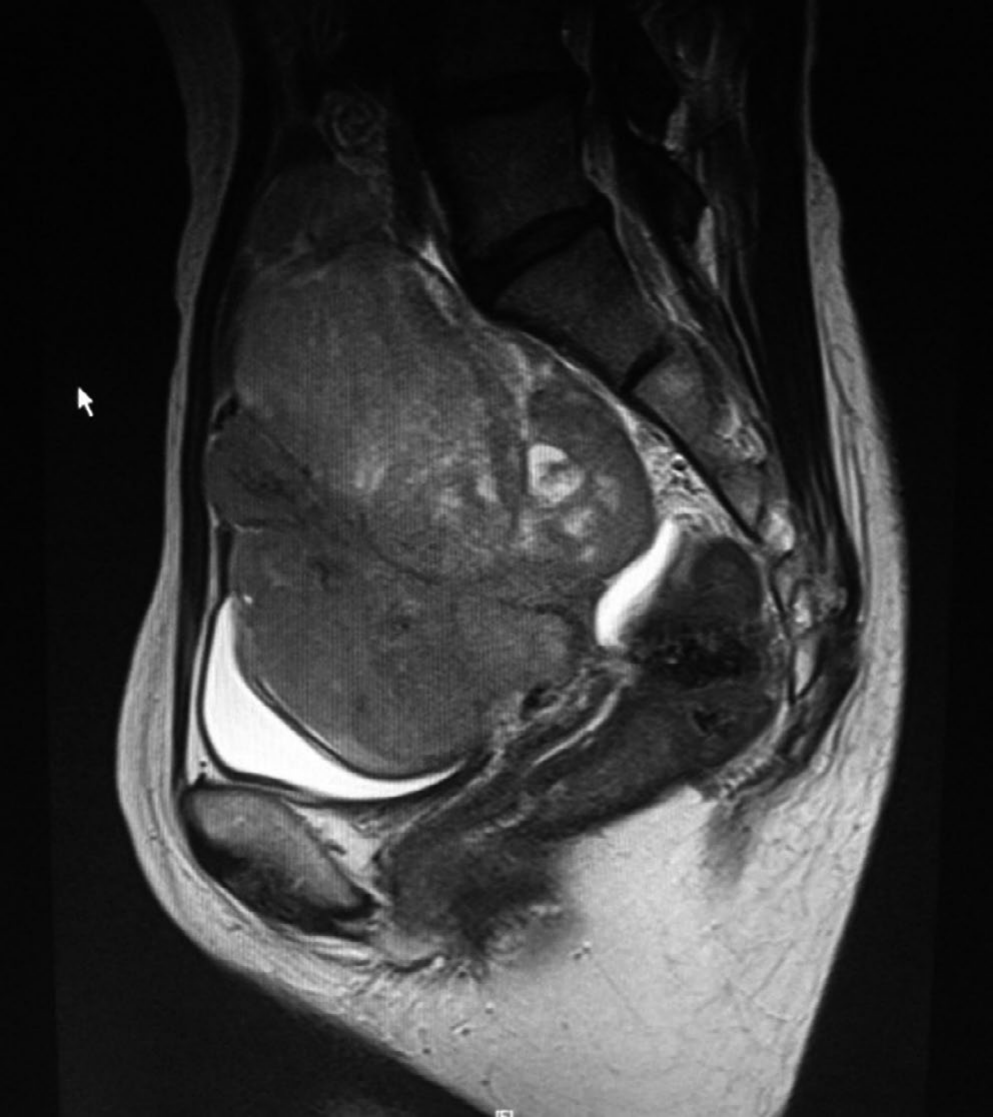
Solid, enhancing right ovarian mass revealed on magnetic resonance imaging
